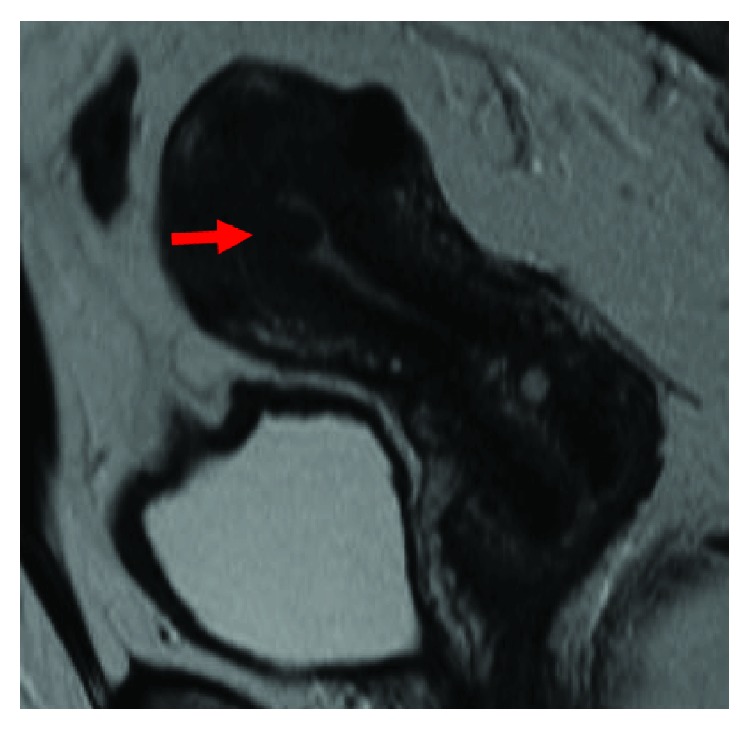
MRI image before operation; T2 weighted, sagittal section image. MRI did not describe the cervical mass, and a 15 mm myoma is located in the uterus (→).
